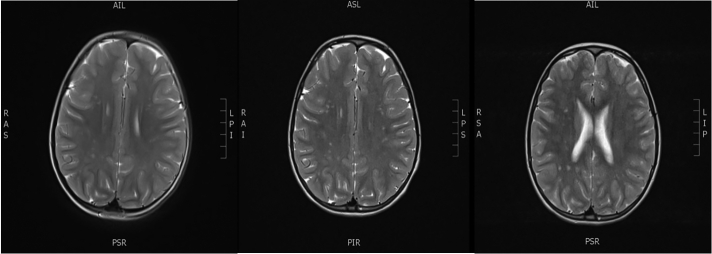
Cerebral MRI (T2-weighted) of the patient at the age of 6 years (A) shows mild abnormalities in centrum semiovale, bilateral subcortical frontal white matter and in the deep frontoparietal white matter. At the age of 9 years (B) progression of nonspecific deep and subcortical white matter signal changes are seen. MRI at the age of 10 years (C) shows no significant changes in previously described hyper-intensive lesions.
